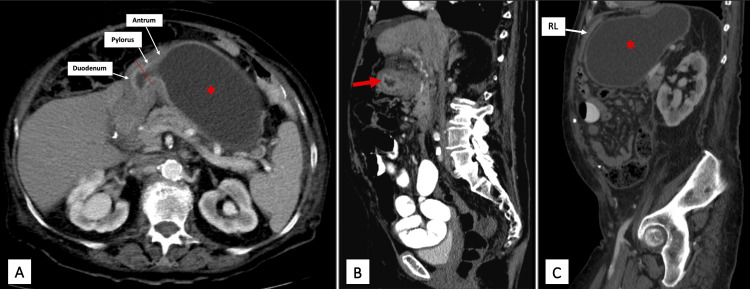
Abdominal CT scan with contrast 1A: Axial view of the distended fluid-filled excluded stomach (*) with contiguous thickening of the antrum, pylorus, and proximal duodenum (arrows). The stenotic pyloric sphincter is bisected by the dashed red line. 1B: Sagittal view showing mucosal thickening at the proximal duodenum (red arrow). 1C: Impingement of the Roux limb (RL) by the distended excluded stomach (*).
